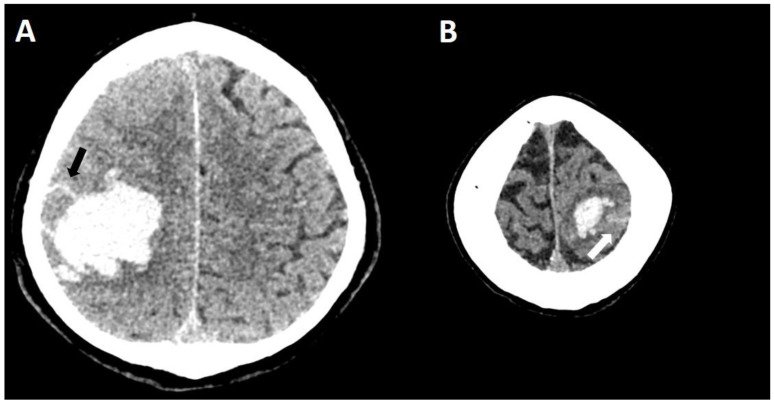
Subarachnoid extension of lobar hemorrhage demonstrated on non-enhanced CT ((A): 100 kV, 596 mAs, slice thickness 1 mm; (B): 120 kV, 320 mAs, slice thickness 1 mm). Leakage of blood into subarachnoid space effacing adjacent sulci (arrows).
